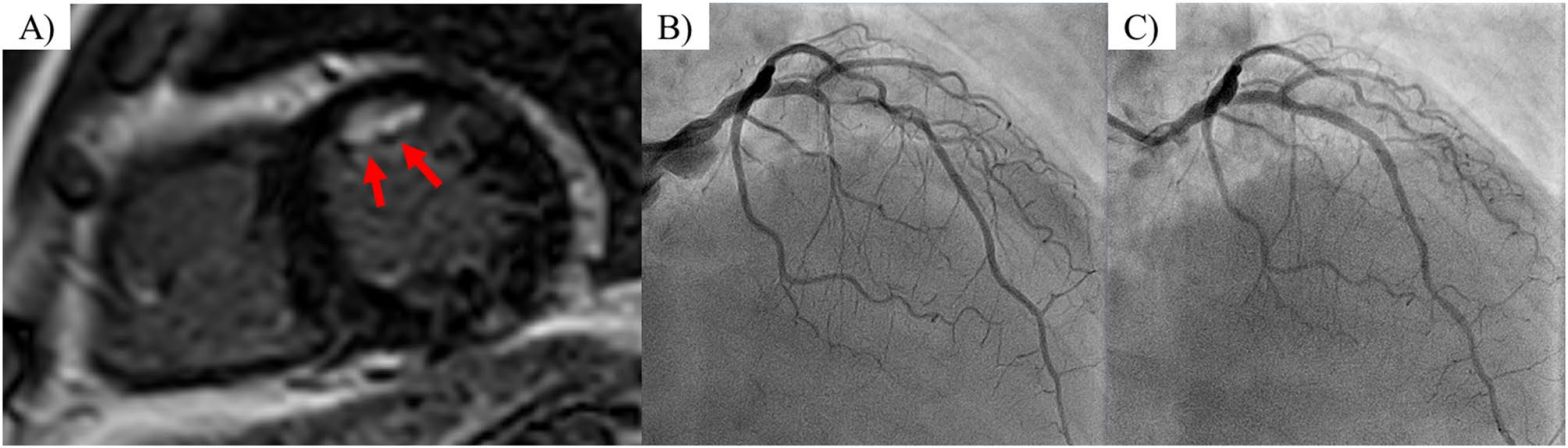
Representative images of patients with unrecognized myocardial infarction. A 69-year-old man with a history of diabetes mellitus with stable coronary artery disease. (A) Short-axis view of delayed-enhancement cardiac magnetic resonance imaging with anterior late gadolinium enhancement (arrowheads). (B) Pre-PCI angiography showing significant coronary stenosis in the proximal left anterior descending artery. The FFR value was 0.53. (C) Post-PCI angiography demonstrating implantation of a drug-eluting coronary stent in the LAD lesion. The FFR value after PCI was 0.91. *PCI* percutaneous coronary intervention, *FFR* fractional flow reserve, *LAD* left anterior descending artery.
